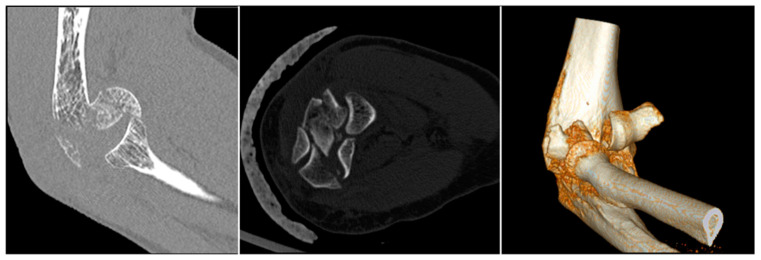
CT scan with 3D reconstruction helps the surgeon detect posterior comminution, analyze the number of fracture fragments, and understand the medial extension of the fracture.
